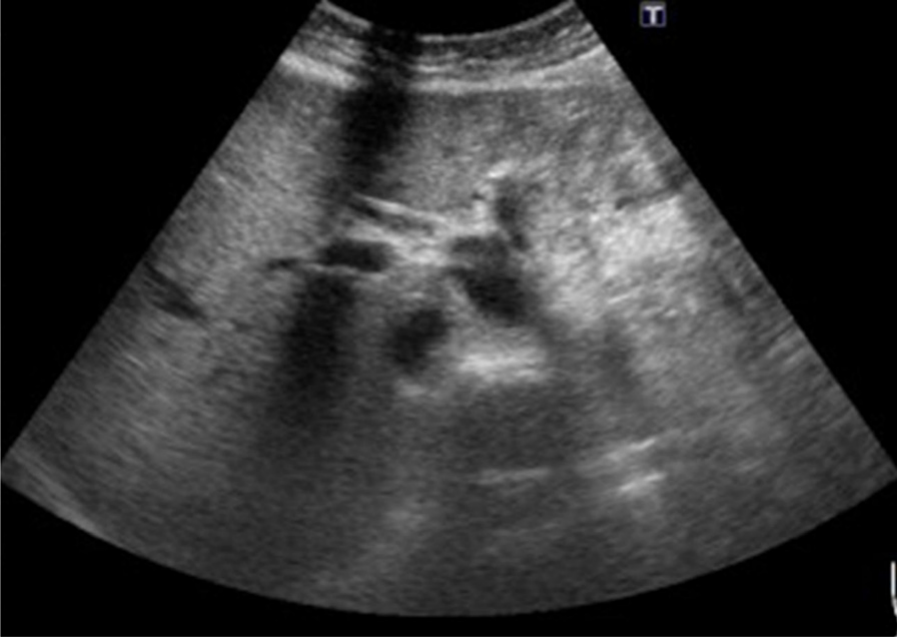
Abdominal ultrasonography. Abdominal ultrasonography (US) showed a multiloculated cystic mass over the lateral segment of left hepatic lobe.
